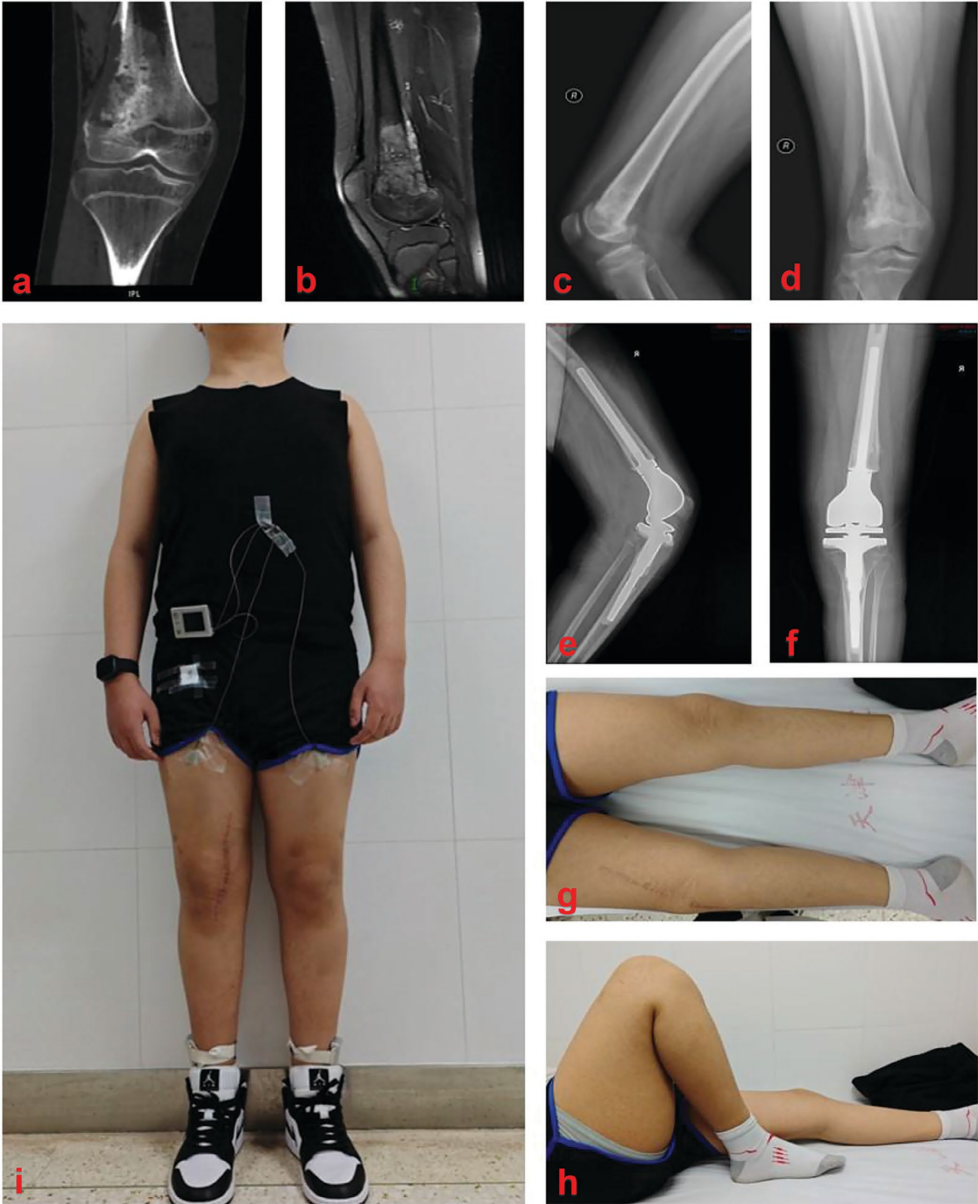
Representative case of an 11‐year‐old boy diagnosed with distal femoral osteosarcoma who underwent RHK (a, b) Preoperative CT and MRI scans of the affected knee; (c, d) Preoperative anteroposterior and lateral radiographs; (e, f) Postoperative anteroposterior and lateral radiographs; (g, h) One‐year follow‐up images showing satisfactory joint motion, stable fixation, and no evidence of prosthetic loosening; (i) Gait analysis via the IDEEA wearable system.
